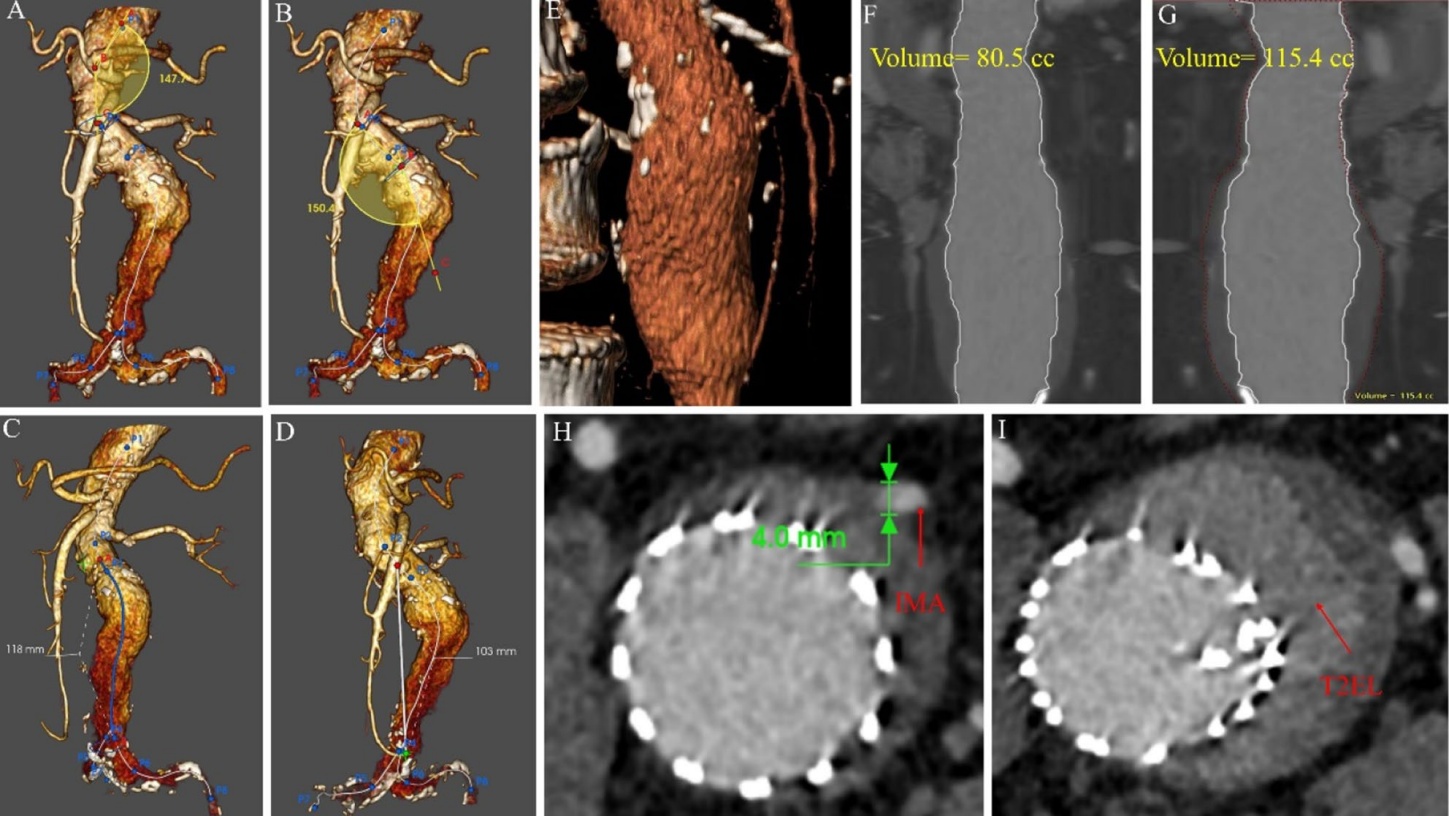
The morphological features of abdominal aortic aneurysm. (A) Angle (α) is defined as the angle between the central line of the upper abdominal aorta of the kidney and the central line of the lower abdominal aortic aneurysm neck. (B) Angle (β) is the angle between the aneurysm neck and the centerline of the aneurysm body. (C) L1 is defined as the length of the central line of the aneurysm. (D) L2 is defined as the length of the distance at the beginning to the end of the aneurysm. (E) The three-dimensional reconstruction of aneurysm sac by 3-Mensio software for estimating the proportion of thrombus. (F) The volume of blood in the aneurysm sac. (G) The volume of the whole aneurysm sac. (H) The diameter of the inferior mesenteric artery was measured by Endosize. (I) The occurrence of T2EL was identified by CTA.
